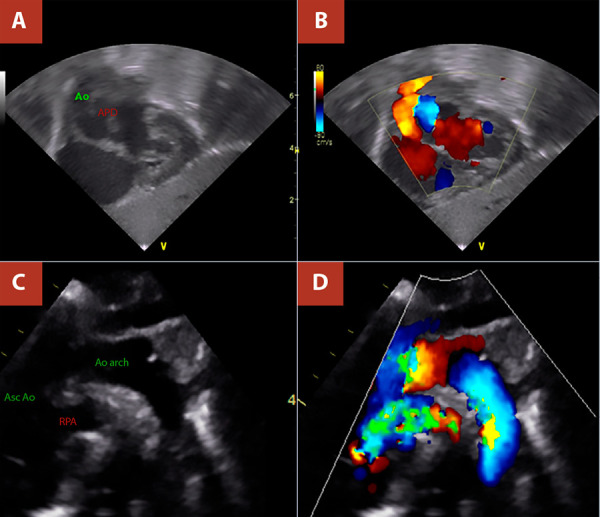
Transthoracic echocardiography, Case 1. A) and B) Subcostal views showing the origin of the right pulmonary artery from the ascending aorta. C) and D) Suprasternal views demonstrating the origin of the right pulmonary artery from the posterior wall of the ascending aorta. RPA: right pulmonary artery.
